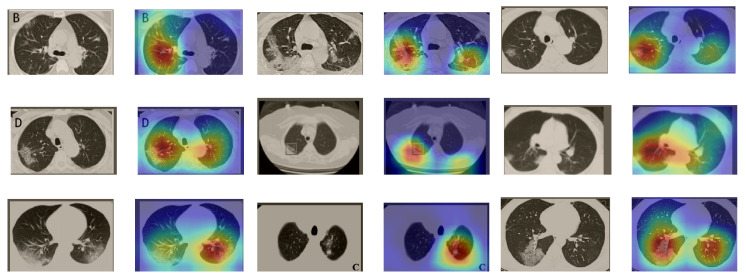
Grad-CAM visualizations for sample CT images from the COVID19-CT dataset. Our DenseNet169 model correctly classified them as COVID-19 cases and highlighted the most relevant regions, as shown in the corresponding localization maps.
